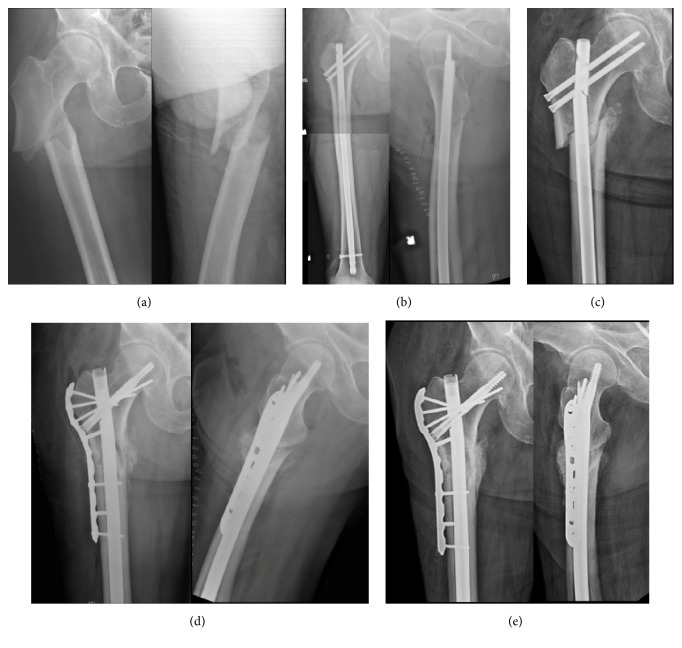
(a) Atypical right subtrochanteric femur fracture created in a 68-year-old male with 8-year history of bisphosphonate use. (b) He underwent open reduction internal fixation with a locked piriformis reconstruction nail within 24 hours of injury. Note anatomic alignment immediately postoperatively. (c) At 8 weeks postoperatively, he had increased pain in his hip and felt a pop. New radiographs reveal hardware failure with nail fracture and varus collapse. (d) Given his adequate proximal femoral bone stock, he underwent revision open reduction internal fixation with removal of hardware, locked cephalomedullary nailing, and augmentative plating with autologous bone grafting of the nonunion site. (e) At 6 months postoperatively, he has radiographic signs of union and is pain-free and returned to all previous activities.
